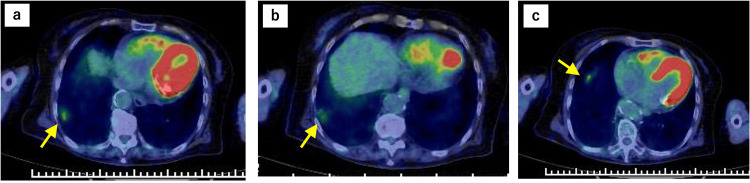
Pre-second SBRT imaging showing new pulmonary metastatic lesions (a, b) PET-CT showed new lesions in the vicinity of the S9 lesion in the right lung and (c) a new metastatic lesion in the S4 of the right lung SBRT: stereotactic body radiotherapy; PET-CT: positron emission tomography-computed tomography
